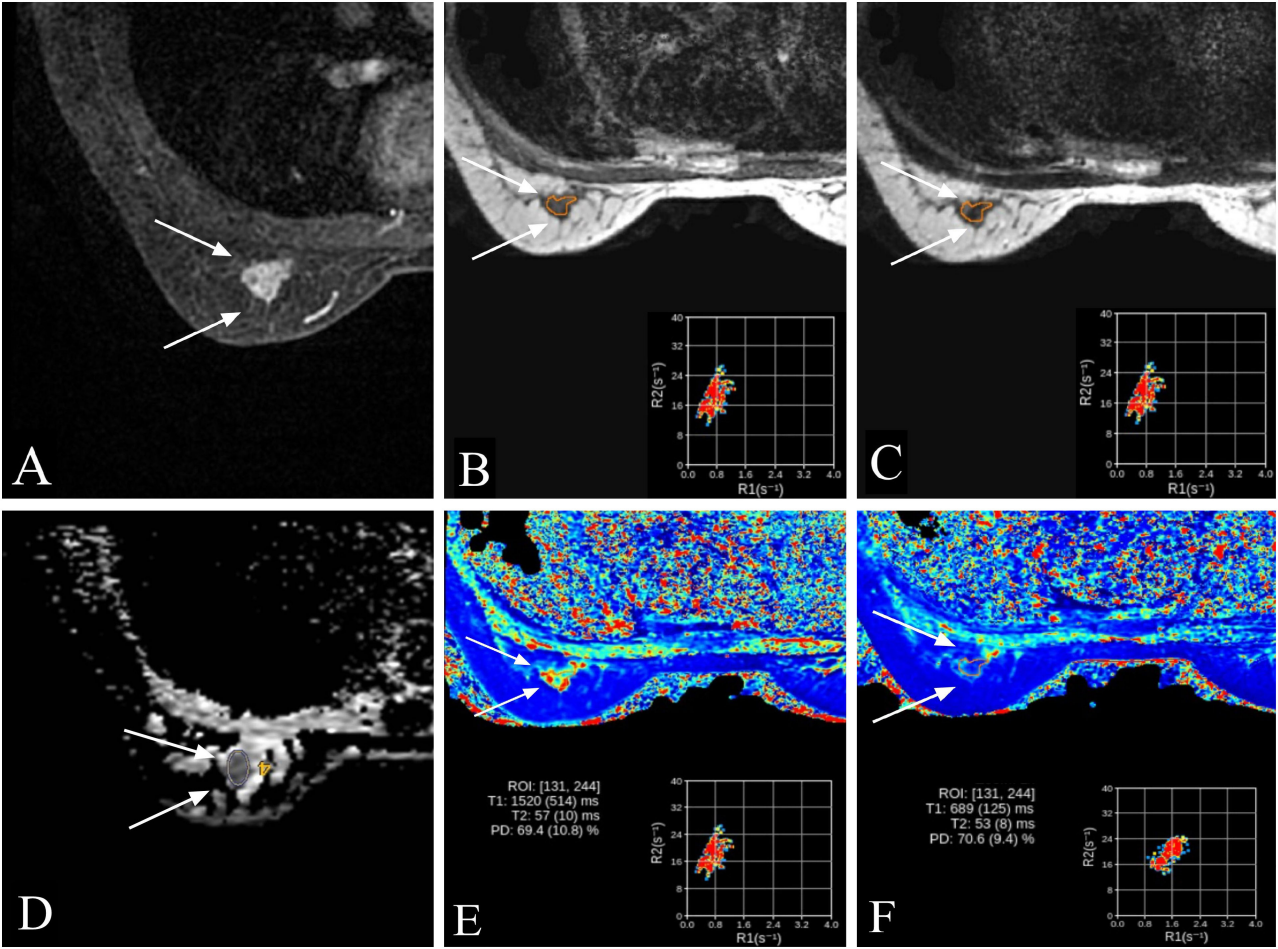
Representative multiparametric MRI findings in a 52-year-old woman with invasive breast cancer and HER2-low expression (IHC 1+). (A) DCE-MRI showing a moderately enhancing lesion in the right breast (arrows). (B, C) SyMRI parametric maps (T1-pre and T2-pre) with ROI placement. (D) ADC map from MUSE-DWI demonstrating restricted diffusion (arrows). (E) Pre-contrast SyMRI T1 mapping (T1-pre) with quantitative measurement. (F) Post-contrast SyMRI T1 mapping (T1-Gd) showing shortened relaxation time within the lesion.
